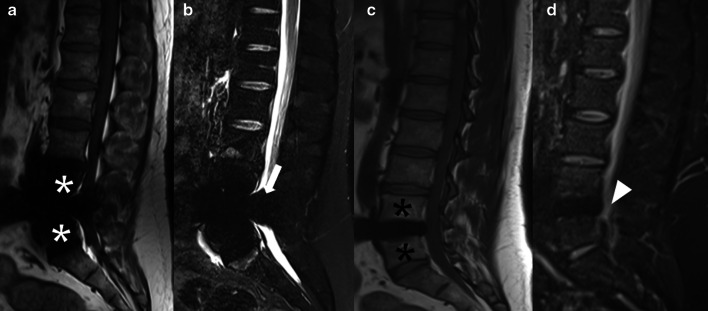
Technique for metal artifacts reduction after a disc replacement procedure at the L4-5 level. Sagittal T1-weighted (a) and STIR (b) MR images show susceptibility artifacts due to the metallic disc prosthesis, limiting the evaluation of the vertebral bodies (white asterisks) and spinal canal (arrow) from L3-4 to L5-S1 levels at these levels. Sagittal T1-weighted (c) and STIR (d) MAVRIC sequences to reduce metal artifacts improve the evaluation of the L3-4 and L5-S1 levels (black asterisks), and the spinal canal (arrowhead) and posterior elements
